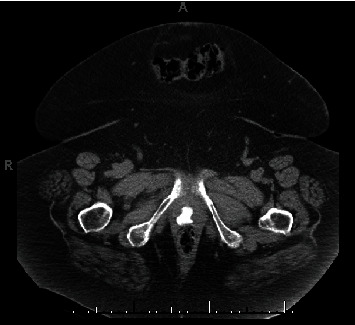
Axial CT scan image of the pelvis showing 2.16 × 1.76 cm calcified vaginal mass.
